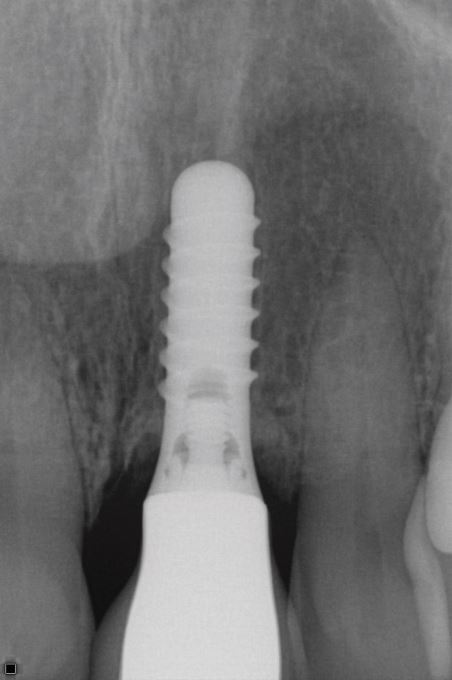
Intraoral periapical radiograph of 21 showing apparent good radiographic bone level
